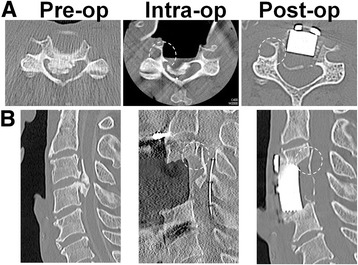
Pre-op*, intraoperative (*Intra-op*), and *Post-op* CT images in the axial plane (a) and sagittal plane (b)
